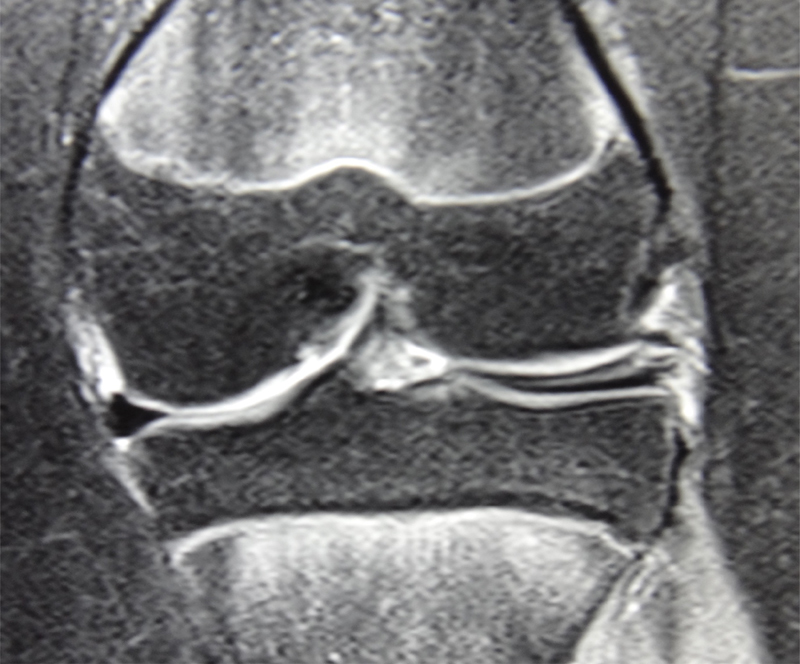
Coronal slice of MRI showing discoid lateral meniscus with complex horizontal tear. MRI, magnetic resonance imaging.
